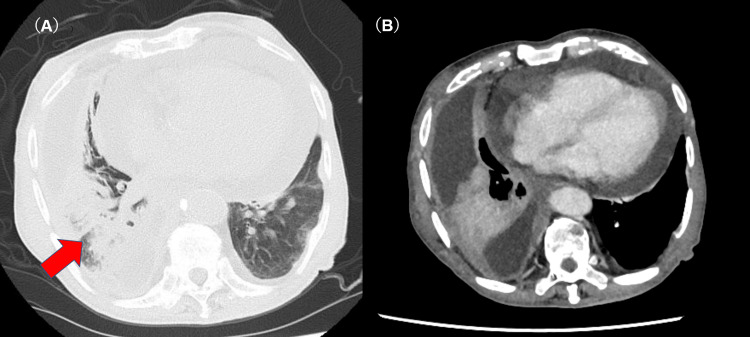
Chest CT images (A) An axial chest CT image shows an infiltrating shadow in the right lower lobe (arrow), right pleural effusion, and pleural thickening on the mediastinal side. (B) A contrast-enhanced axial CT image of the chest shows pericardial effusion.
